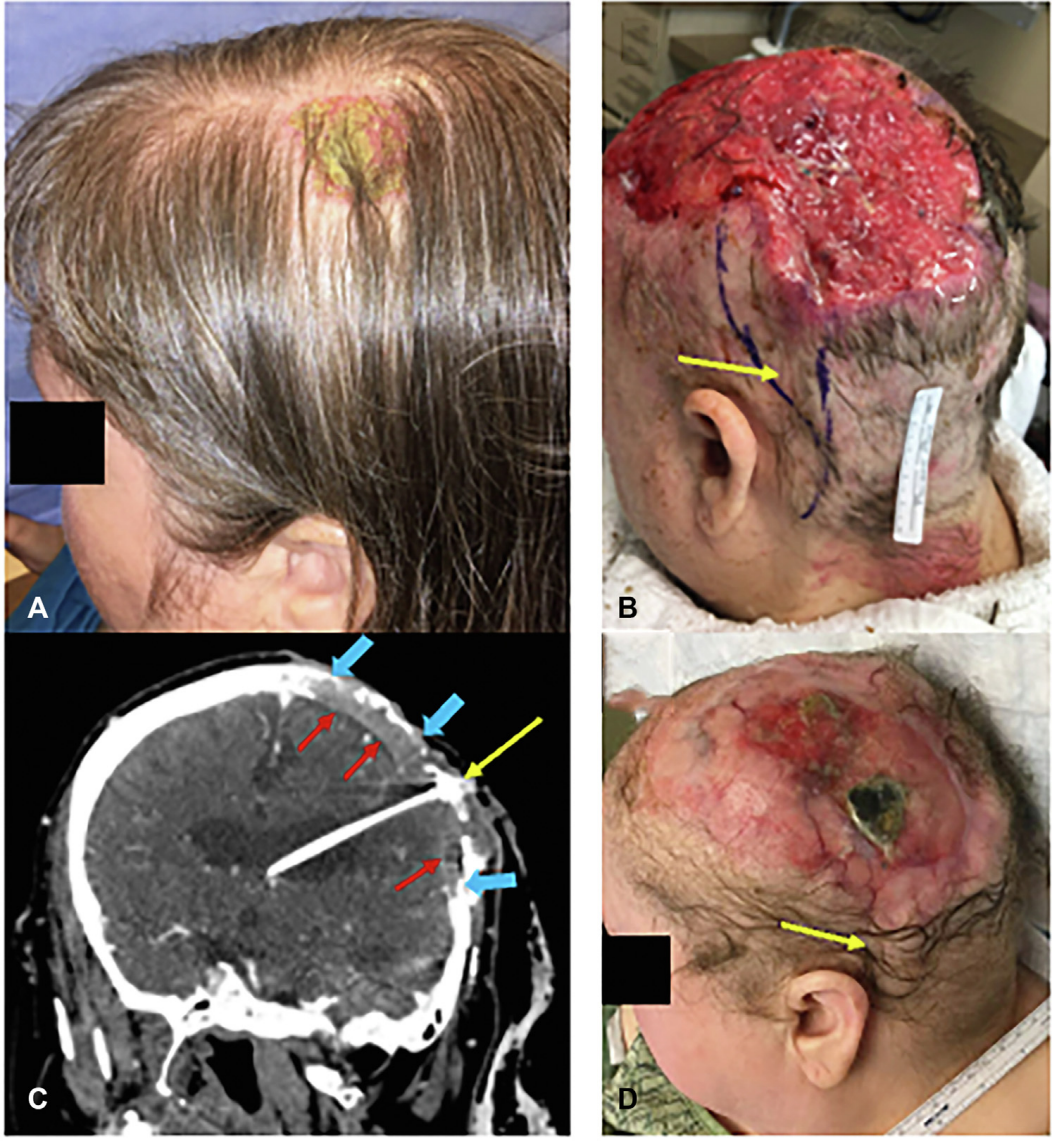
Cutaneous squamous cell carcinoma (CSCC) of the scalp in a spina bifida patient with a ventriculoperitoneal shunt (VPS) and a renal transplant on immunosuppression. (A) Photograph taken immediately prior to left scalp biopsy, which showed CSCC *in situ* (CSCCIS*)*, taken 6 years prior to (B), before any treatment. The CSCCIS was treated with 5-fluorouracil (5-FU) cream, but development into CSCC necessitated Mohs surgery for the invasive component, with the *in situ* component treated with additional 5-FU cream. (B) The patient did not follow up for several years and then presented with a 13 cm ulcerated plaque on the left scalp overlying the prior CSCC site. The outline of the retroauricular portion of the subcutaneous VPS catheter was visible and palpable (*yellow arrow*), extending from the ulcer to the neck. Biopsy at least 3 cm away from the shunt (white dashed circle) confirmed invasive CSCC with perineural invasion. (C) Computed tomography (CT) scan of the head showed the VPS catheter (*yellow arrow*) surrounded by extensive calvarial destruction (*blue arrows*) and dural involvement (*red arrows*) by the CSCC. The neurosurgery team determined the CSCC was inoperable due to the extent of osseous and dural involvement. (D) The patient underwent multiple lines of systemic therapy over the next 13 months, with CSCC response evident (*yellow arrow*) after carboplatin/paclitaxel.
